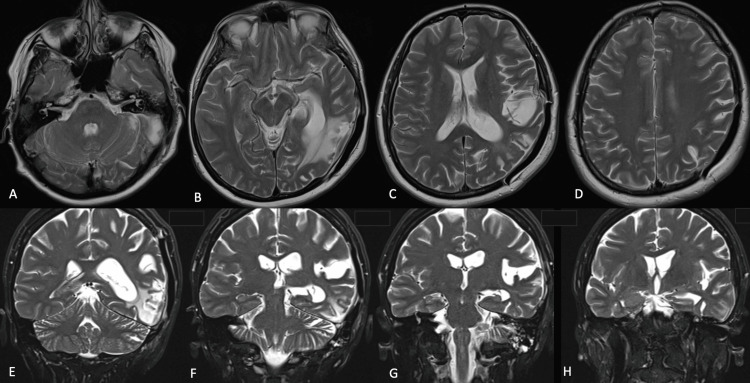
Postoperative T2 turbo spin-echo MRI images in the axial (A-D) and coronal (E-H) planes demonstrating a CSF-filled postoperative cavity in the left temporo-occipital region (B, C), surrounded by gliotic changes (B, E) and associated ex vacuo dilatation of the adjacent temporal horn (B, E). No residual or recurrent lesion is identified.
